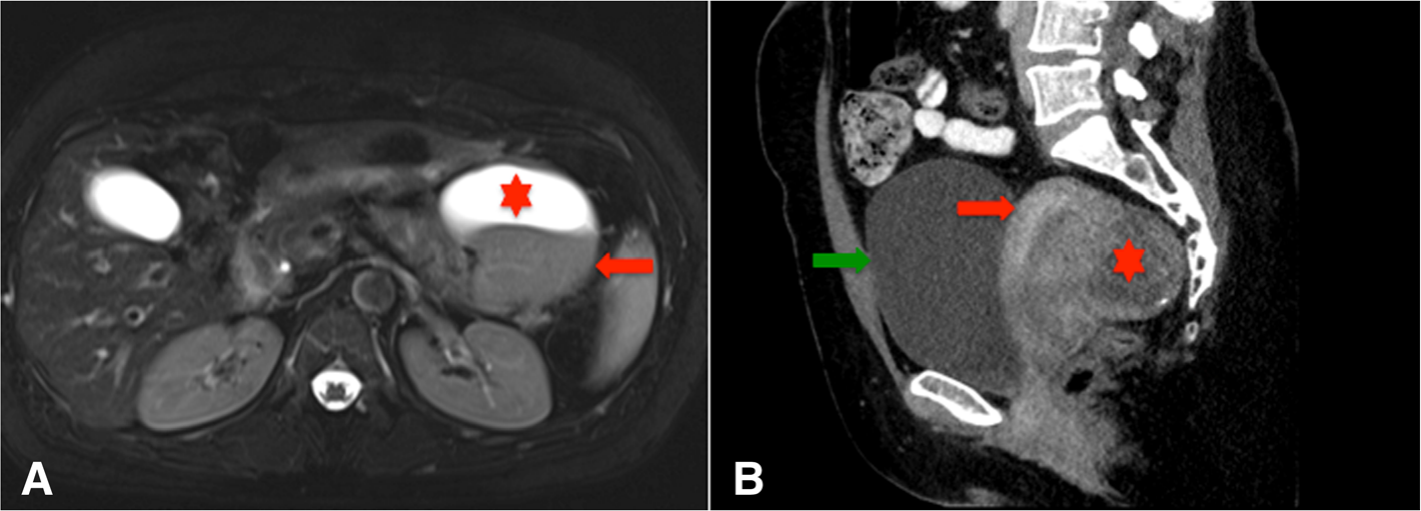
a (T2 axial image, MRI abdomen/pelvis); An MRI of abdomen and pelvis disclosed an 8 cm solid and cystic mass in the tail of the pancreas, which had a solid component (red arrow), as well as a hemorrhagic component (red *). b (sagittal image, CT of pelvis); A CT of pelvis confirmed a fibroid uterus, with a solid appearance to the lesions (green arrow, bladder; red arrow, uterus; red *, the largest uterine mass)
